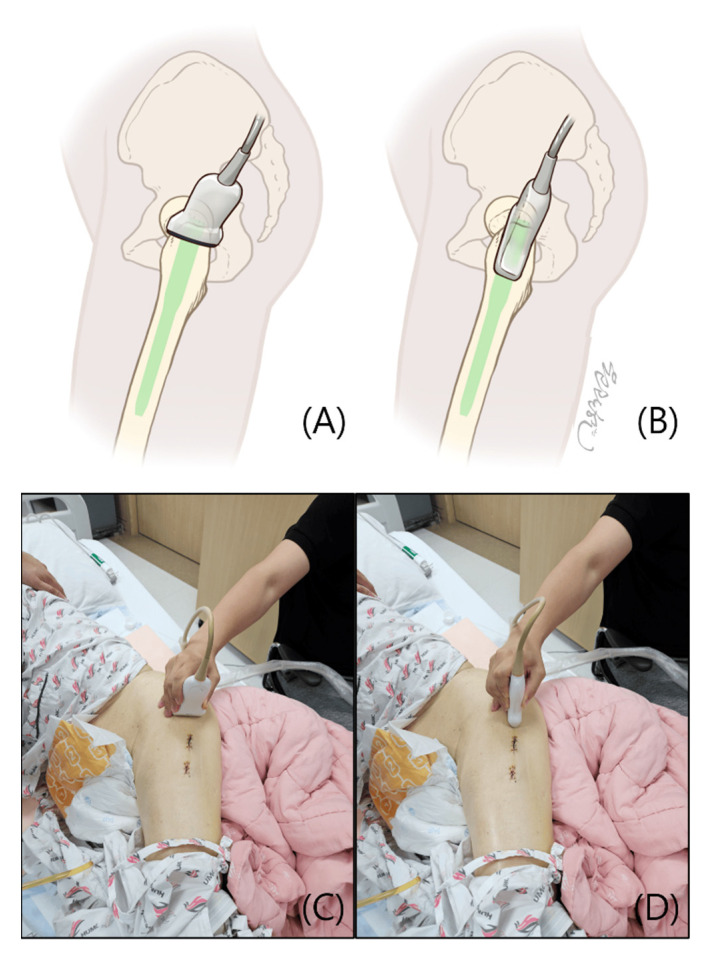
To perform ultrasound, the patient was first positioned in the lateral decubitus position. The hip on the side to be examined was flexed to 15–30° and the knee was flexed to 30°. (A,C) In the short-axis (SAX) view, the transducer was placed on the apex of the GT in a transverse plane perpendicular to the femoral shaft. (B,D) In the long-axis (LAX) view, the transducer was placed on the apex of the GT in a plane parallel to the femoral shaft.
